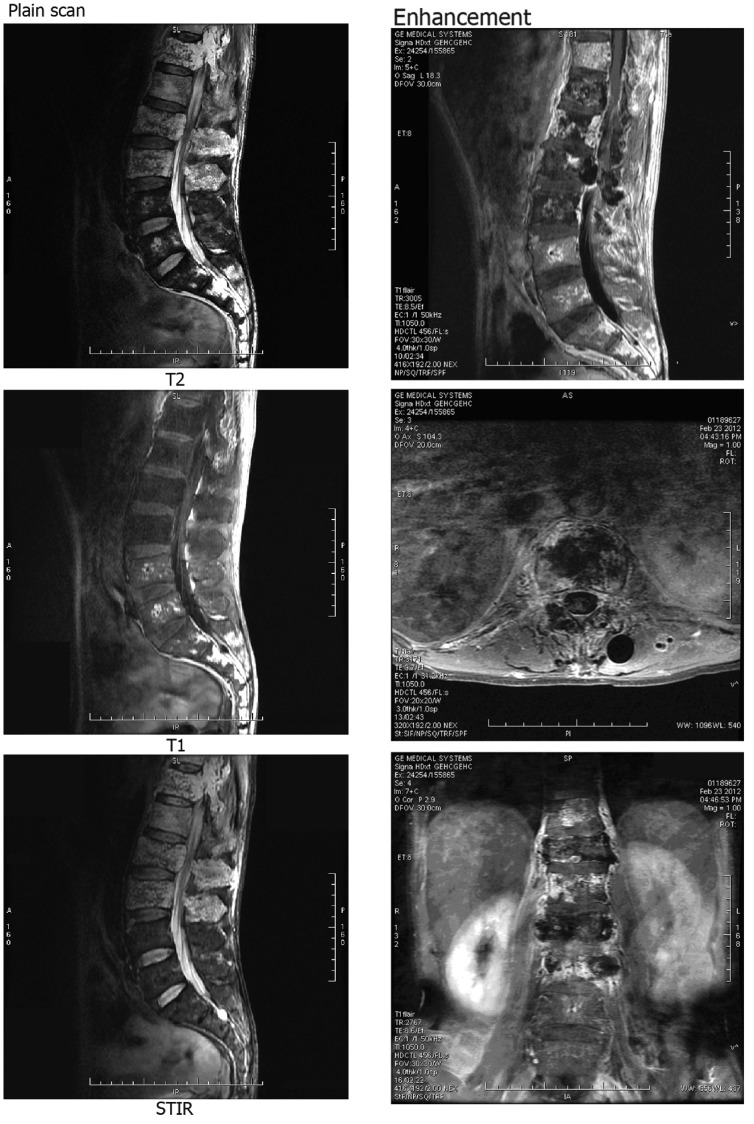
Lumbar vertebrae magnetic resonance imaging (MRI) showing abnormal signals in chest 12-waist 3 vertebral body and spine, with long T1 and long T2 signals. The signal was uneven and the corresponding level back muscle and soft tissue showed mixed T1 and T2 signals, with multiple cystic long T1 and T2 signals. The disc structure appeared normal and the perturbation of the spinal cord and cauda equinal nerve coursed naturally with no abnormal signal in the spinal cord. Enhanced scanning revealed that the lesion exhibited inhomogeneous enhancement and the cystic lesions showed ring enhancement. STIR, short T1 inversion recovery.
